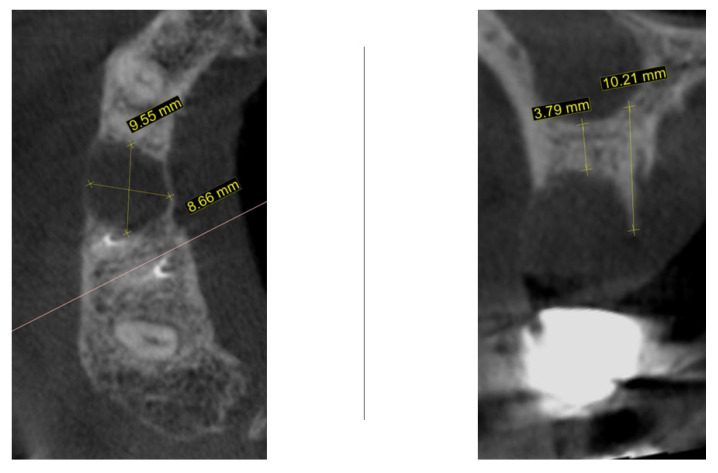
CBCT one month following atraumatic extraction.
